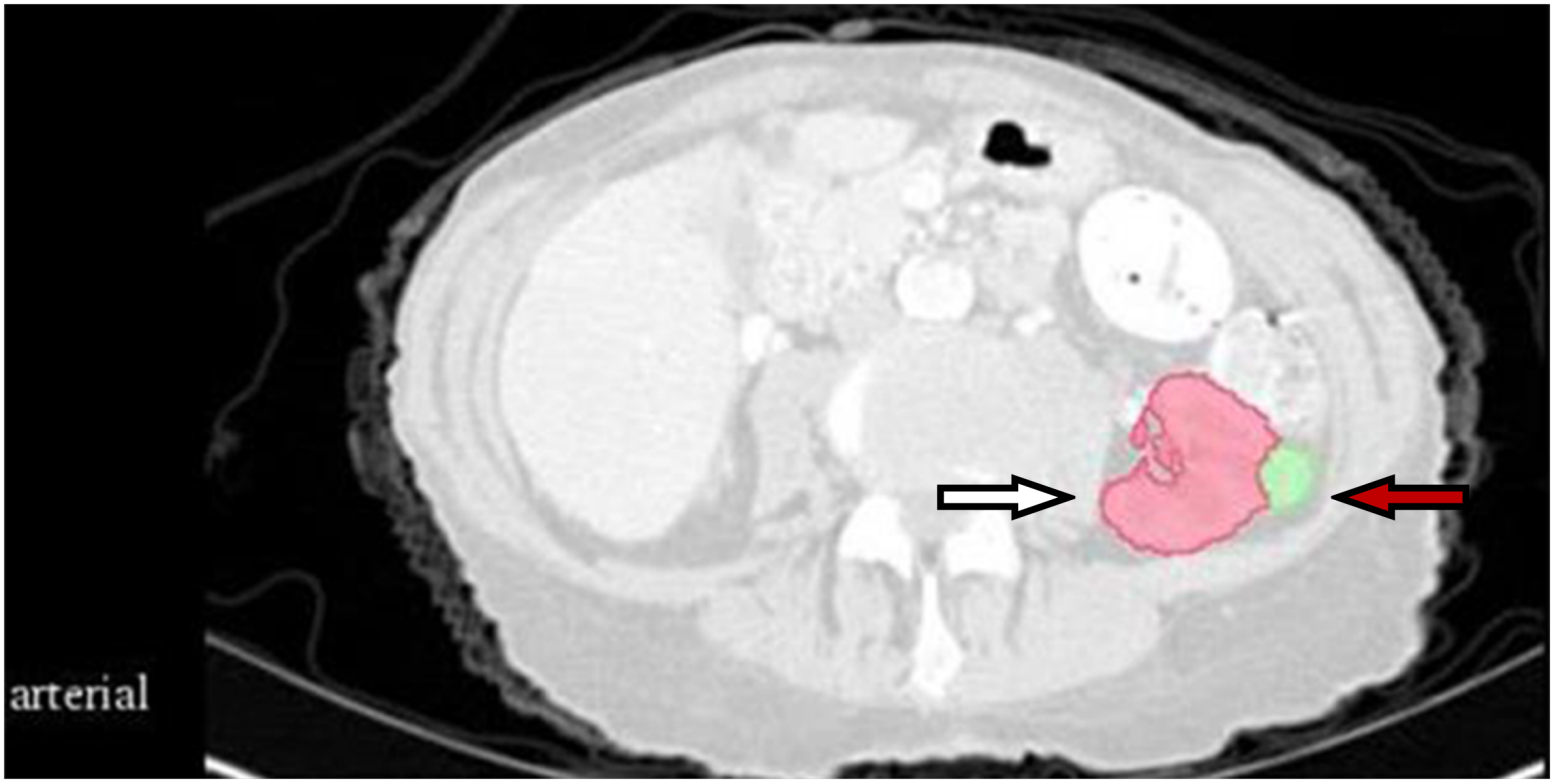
CT section of one patient in the training set. The white arrow indicates normal kidney tissue, and the red arrow indicates gross tumor volume.
